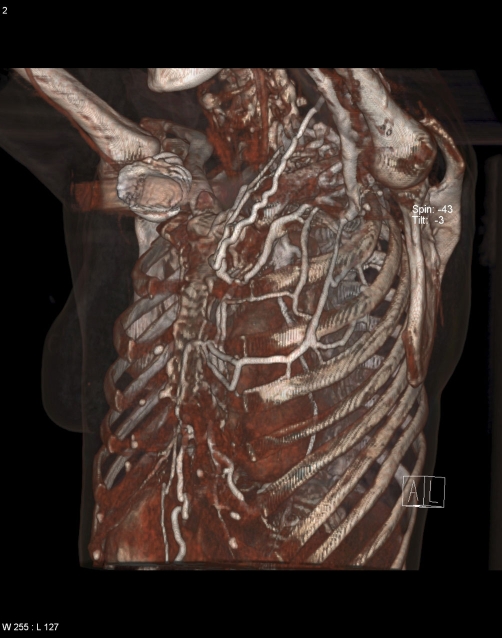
Anterior-posterior (Figure 3, top) and oblique (Figure 4, bottom) three dimensional volume-rendered images from a contrast enhanced computed tomographic scan demonstrating numerous dilated superficial chest veins over the left chest.
